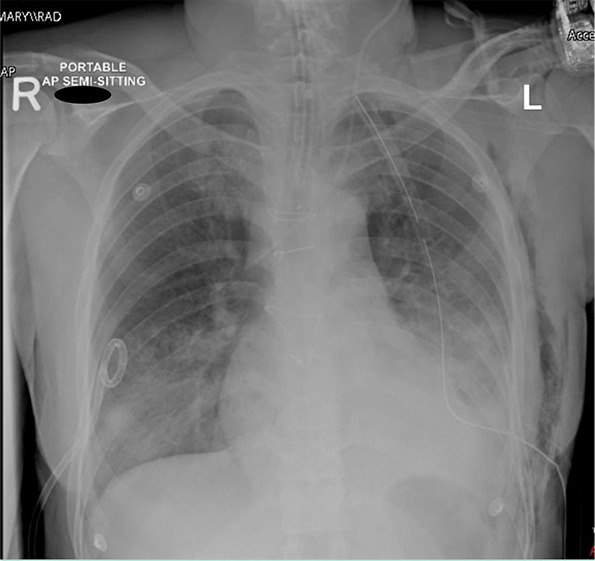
X-ray showing pulmonary edema.
